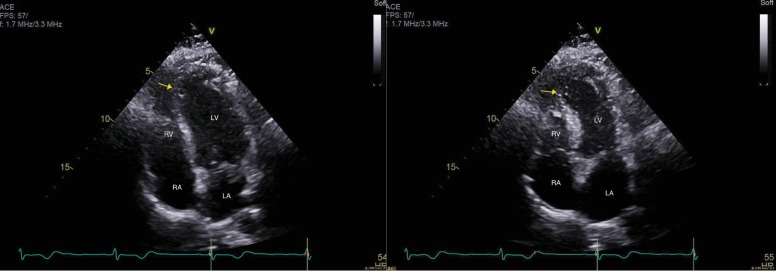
Transthoracic echocardiogram (TTE), revealing a hypokinetic cardiac apex. Yellow arrow on image of diastole (left) and systole (right) shows minimal apical wall motion during the cardiac cycle.
